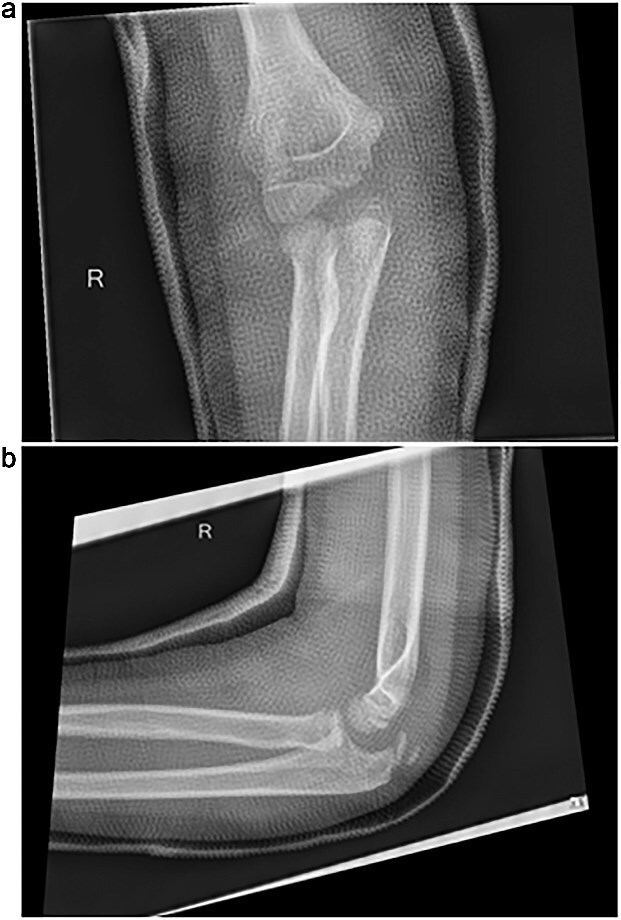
(a, b) Initial radiographs after closed reduction, showing proximal ulna and radial neck fractures.
